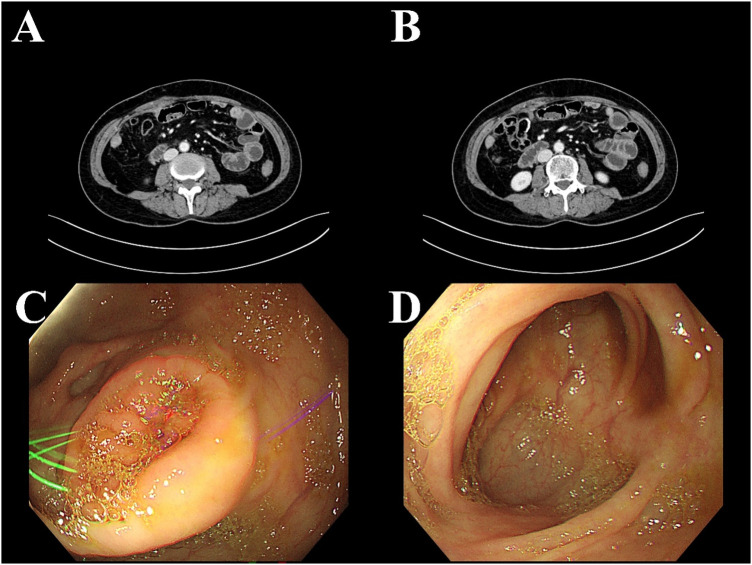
Post-treatment follow-up CT obtained at 48 months. The abnormal venous plexus seen on prior imaging was no longer visible, and lumbar collateral communications were substantially regressed (A,B) (see Supplementary File S8) Colonoscopy showed normal results without obvious abnormalities (C,D).
